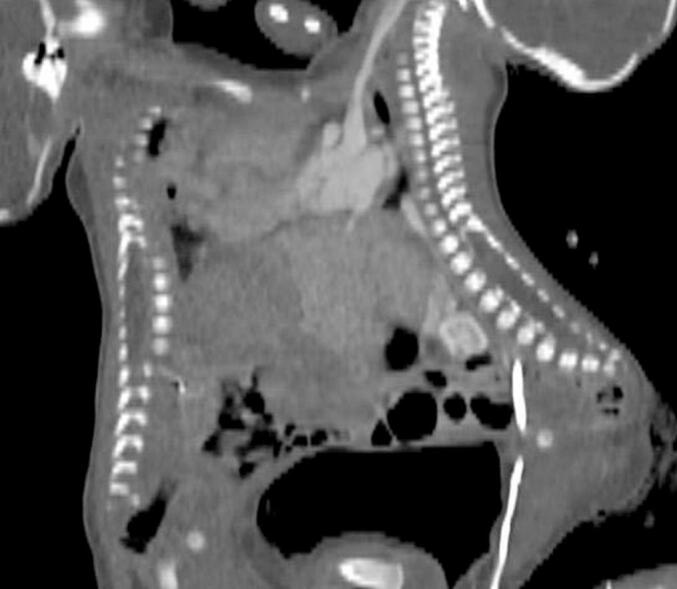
A contrasted CT scan in sagittal views with twin A on the left and twin B on the right in the delayed phase. It shows differential enhancement of the liver parenchyma of both twins.
